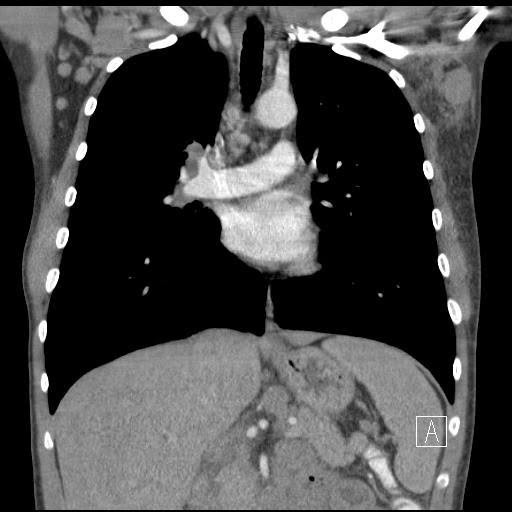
CT scan showing axillary lymph nodes.
